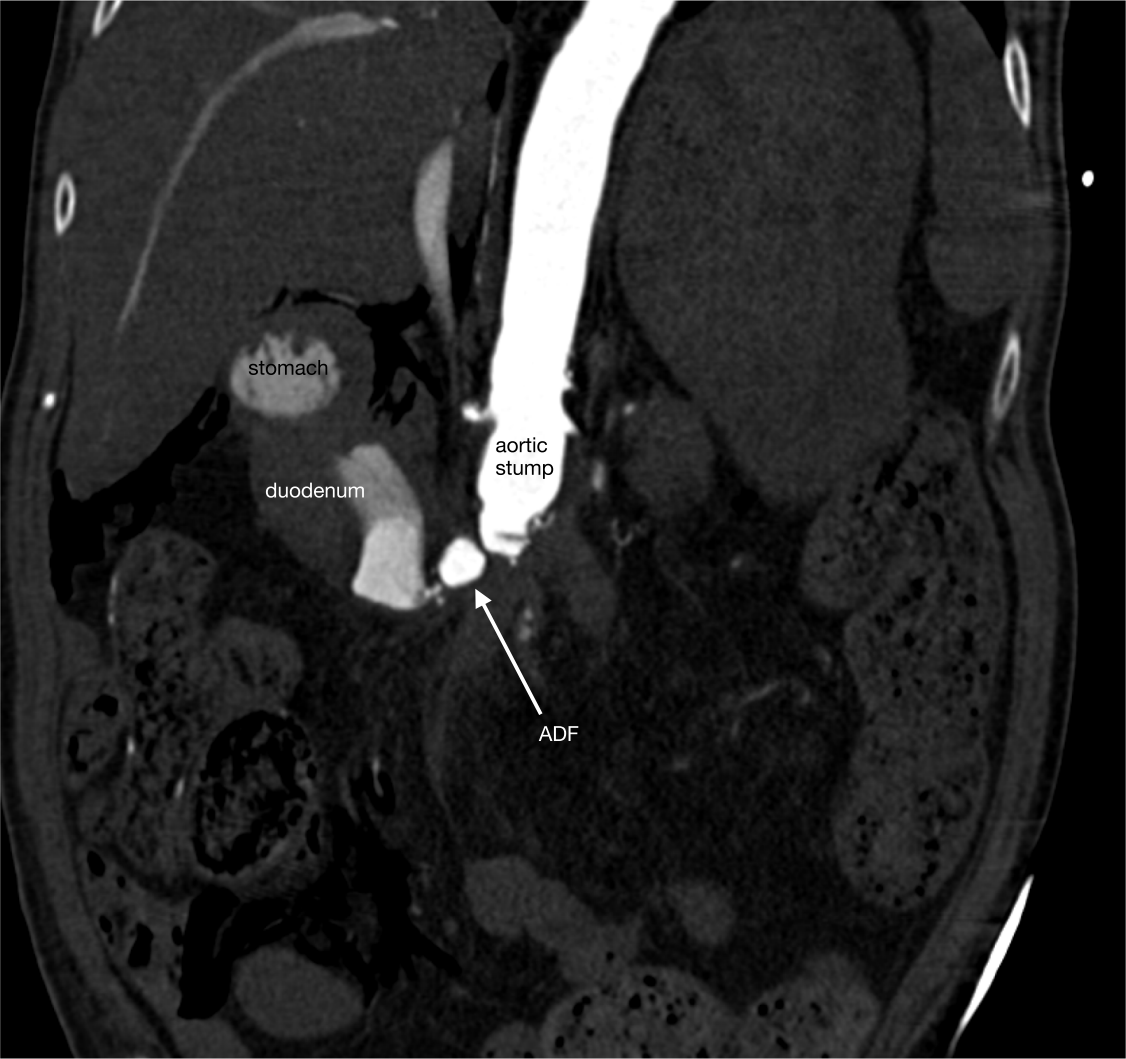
CTA abdomen showing massive extravasation of blood due to a secondary ADF CTA demonstrating massive extravasation of blood through the aortic stump into the duodenum, due to a secondary ADF (marked with an arrow).
